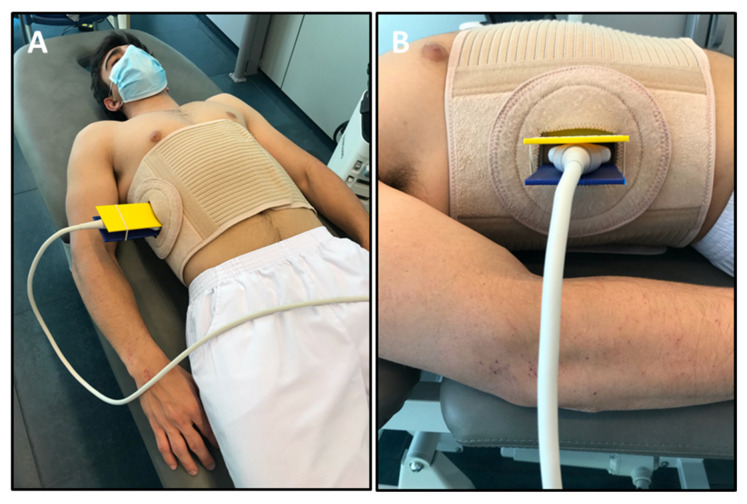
Thoracic orthotic device to measure diaphragm thickness. (A) Thoracic orthosis with the holding device to fix the ultrasound (US) probe. (B) Support and fixation adapter to place the linear US probe perpendicular to the last intercostal space along the mid-axillary line.
